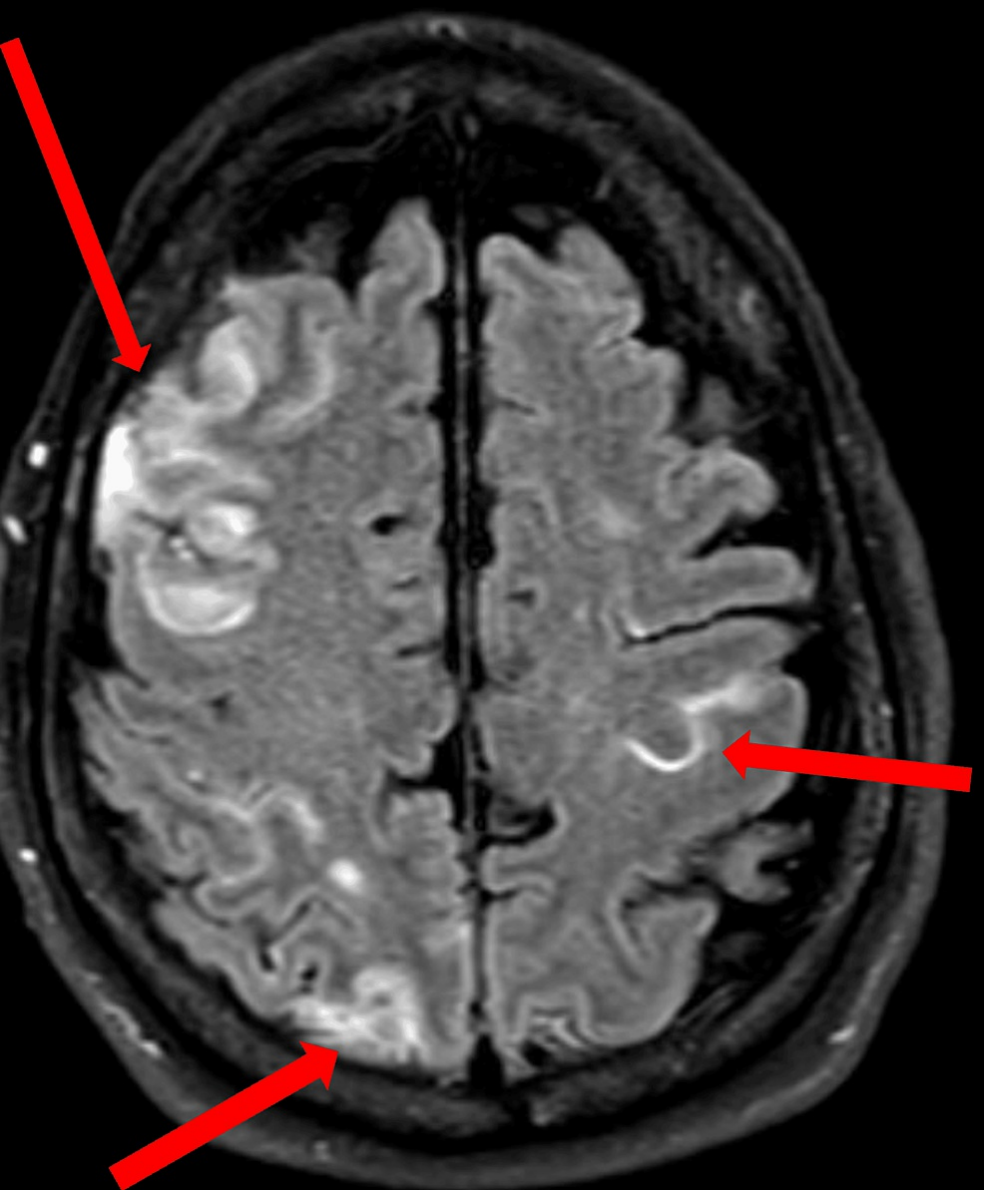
A post-contrast fluid-attenuated inversion recovery (FLAIR) sequence MRI of the brain shows subarachnoid hemorrhage with areas of cortical and leptomeningeal enhancement consistent with cerebritis, subarachnoid hemorrhage, and meningitis (arrows).
